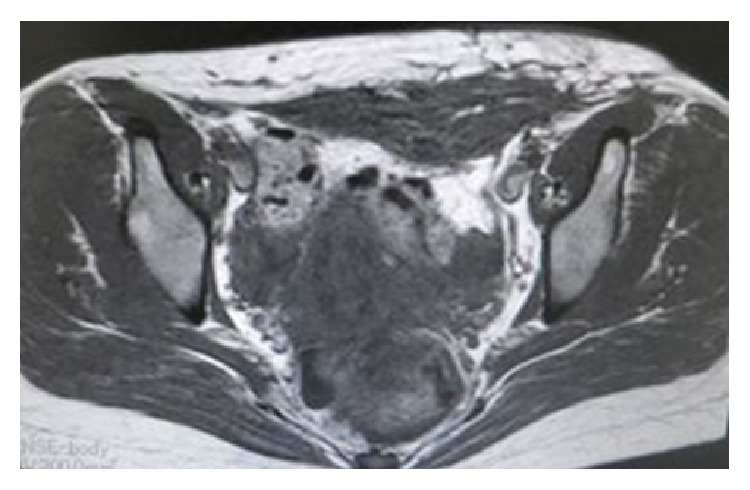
An MRI scan at one postoperative month showed that the rectus abdominis muscle defect had been reconstructed with the vastus lateralis muscle flap.
